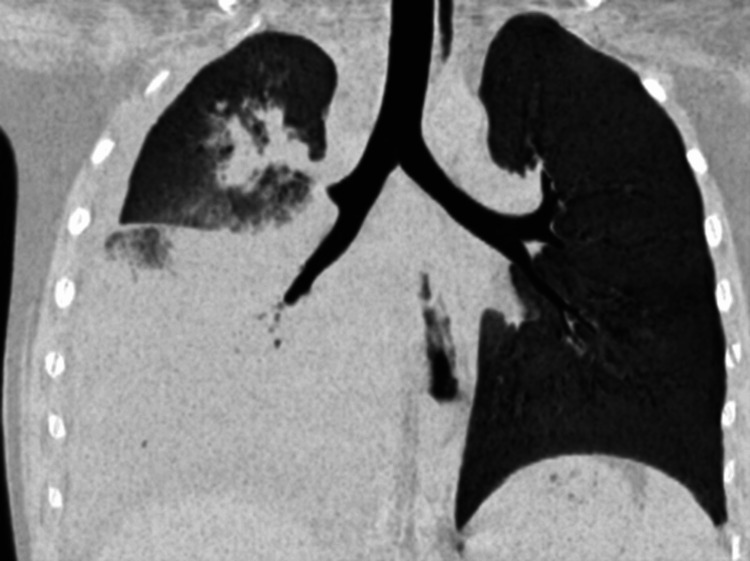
Chest CT scan demonstrates bilateral infiltrates, consolidation of the lower lobe of the right lung and right pleural effusion. CT, computed tomography
